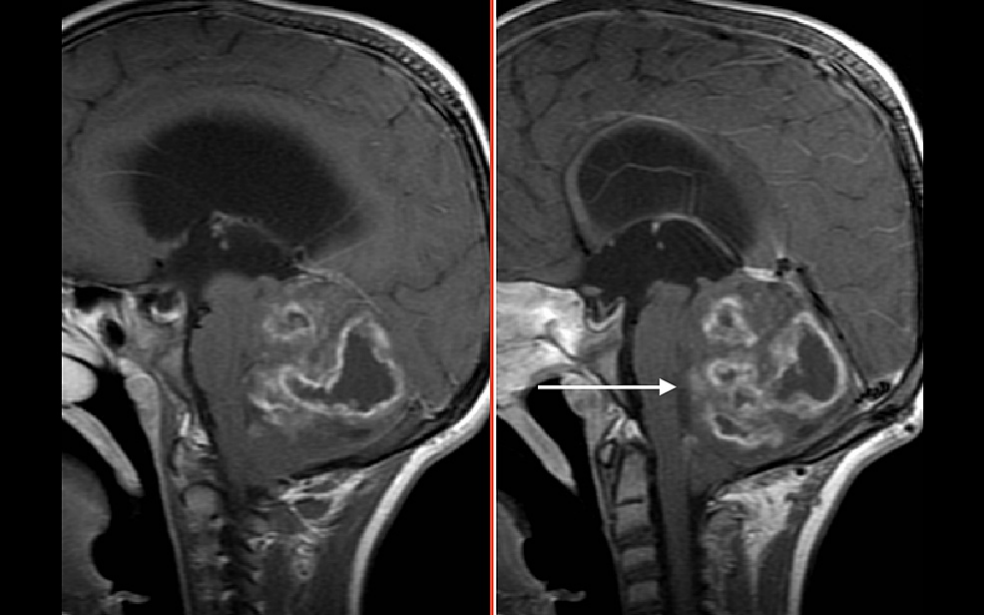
MRI of the brain Sagittal T1 image with contrast showing the tumor compressing the fourth ventricle (white arrow) and the brain stem
